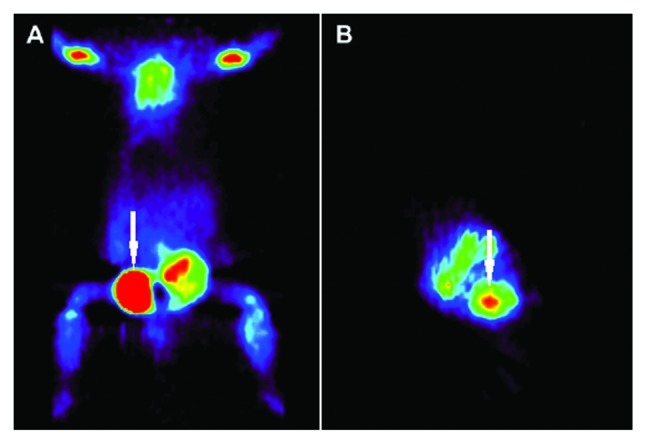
PET-CT scan of the tumor. PET-CT images of subcutaneous neoplasm. (A) Longitudinal CT section and (B) transverse CT section. The tumor is shown as a red-pinkish-colored small ball (arrows).
